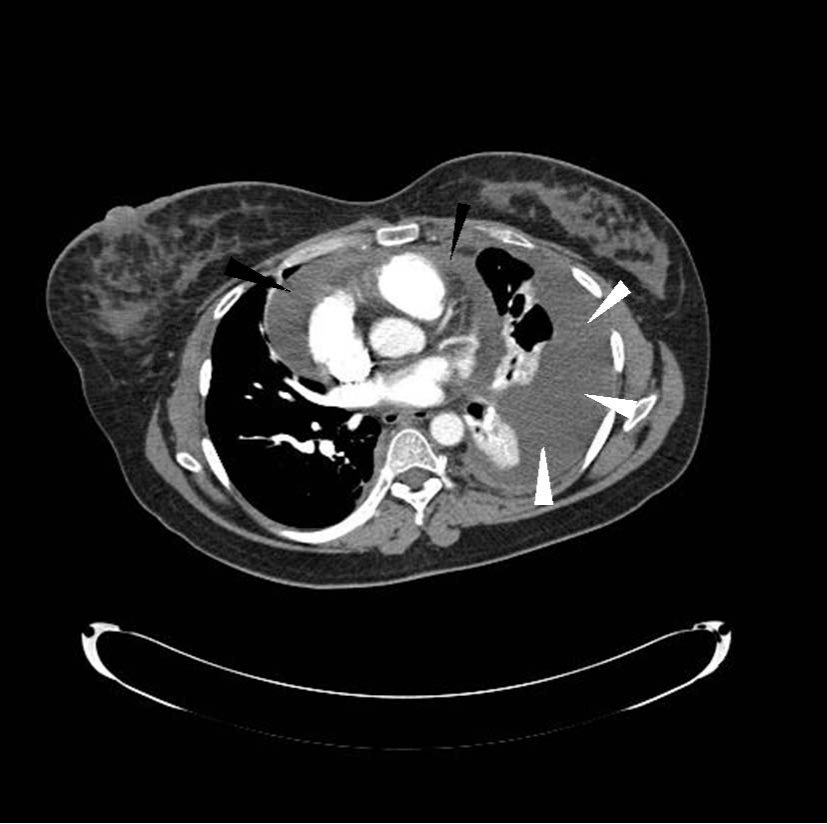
Computed tomography angiogram of the chest. A CT angiogram of the chest performed on hospital day one shows a large pleural fluid accumulation in the left pleural space (white arrowheads) with compressive atelectasis of the left lung as well as a pericardial effusion (black arrowheads). No pulmonary embolus was seen. The pleural fluid was later evacuated and found to be chylous.
